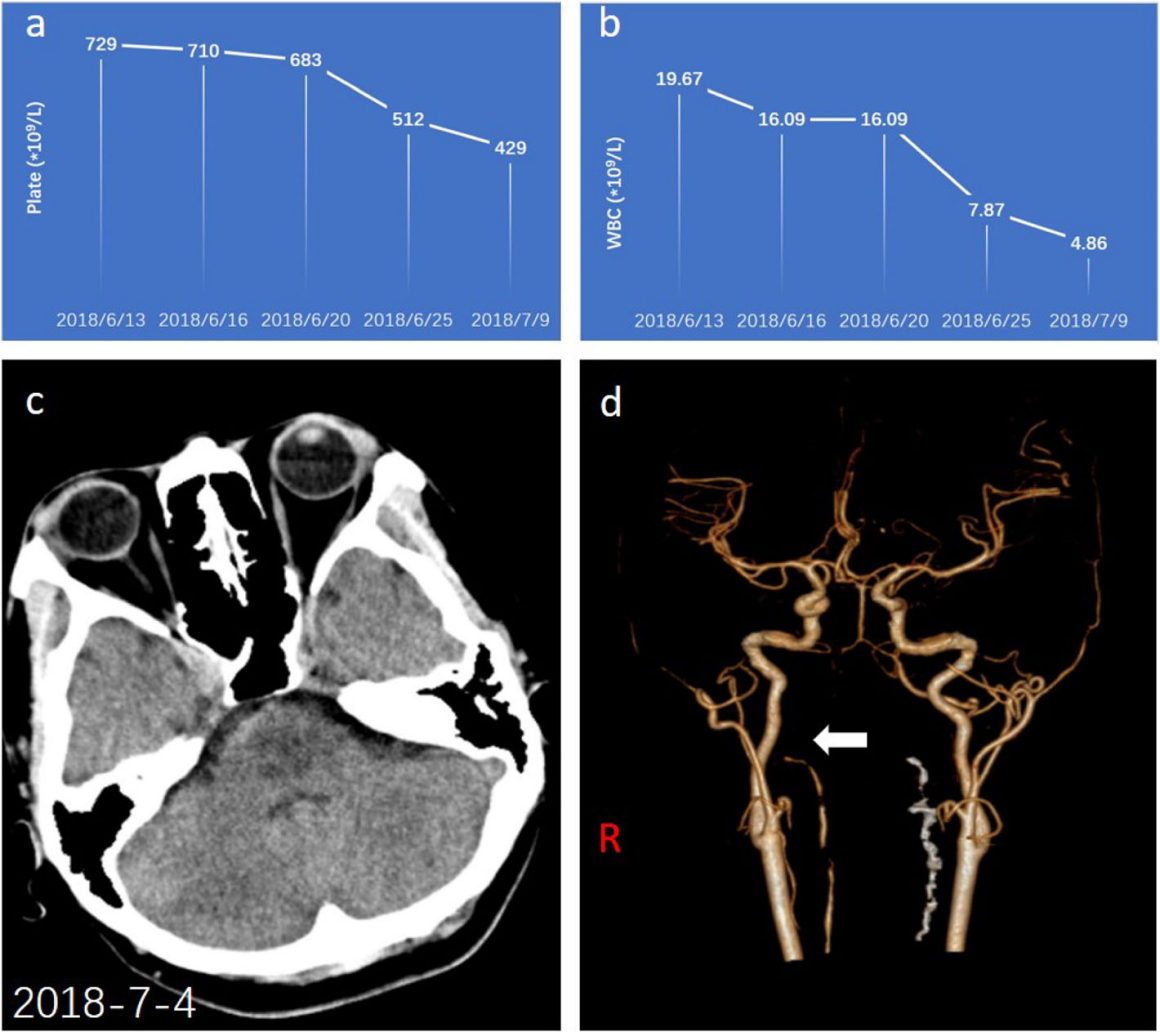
Variations of WBC and platelet counts during the whole course of treatment (a, b). CT scan after 3 weeks displayed an absence of hemorrhagic lesions (c), and occlusion of the right vertebral artery (d)
